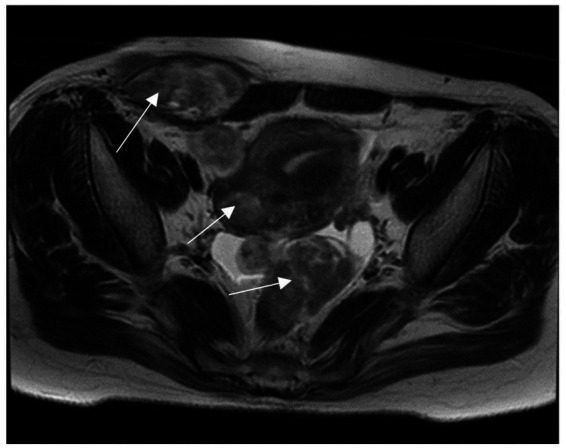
MRI shows the right anterior abdominal wall, myometrium, and multiple pelvic masses.
